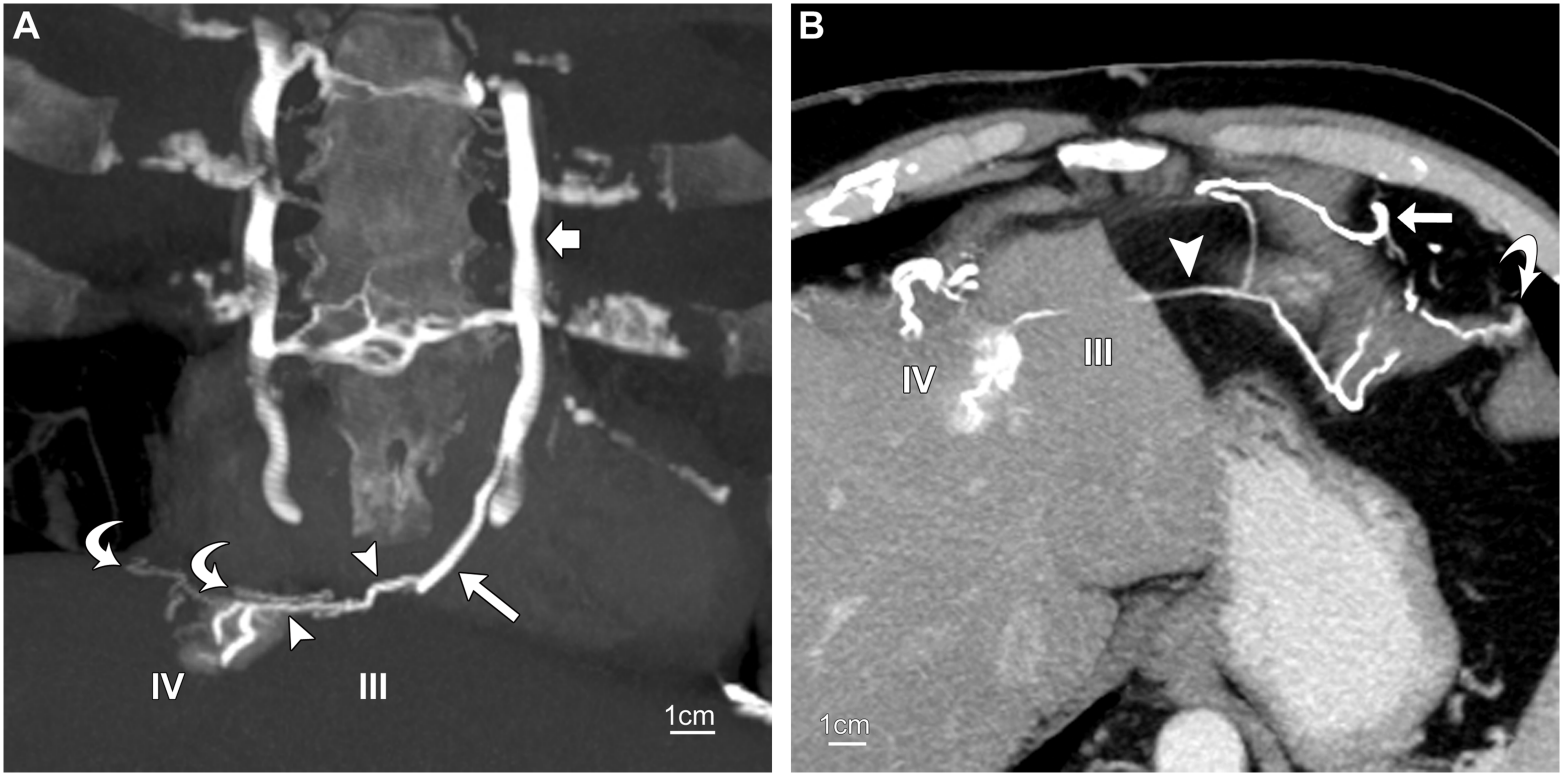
Superior paraumbilical veins. Routine contrast-enhanced CT of chest in a 56-year-old woman with anatomic compression of the left brachiocephalic vein. Slightly oblique coronal 15-mm maximum-intensity-projection (MIP) shows a superior vein (arrowheads) emptying into segment IV at the top of the liver. It communicates with a medial mediastinal branch (long arrow) of the left internal thoracic vein (short arrow) that traverses Morgagni’s foramen and supplies right diaphragmatic veins (curved arrows) as well. (B) Delayed phase contrast-enhanced CT of chest and abdomen in a 57-year-old man with SVC obstruction. Slightly oblique transverse 7-mm MIP shows a superior vein (arrowhead) along the dome of the liver terminating in segment IV. It receives collateral flow from diaphragmatic branches of a left anterior mediastinal vein (straight arrow) in the cardiophrenic fat pad and the left pericardiacophrenic vein (curved arrow). The mediastinal vein descended in the anterior mediastinum from the left brachiocephalic vein.
